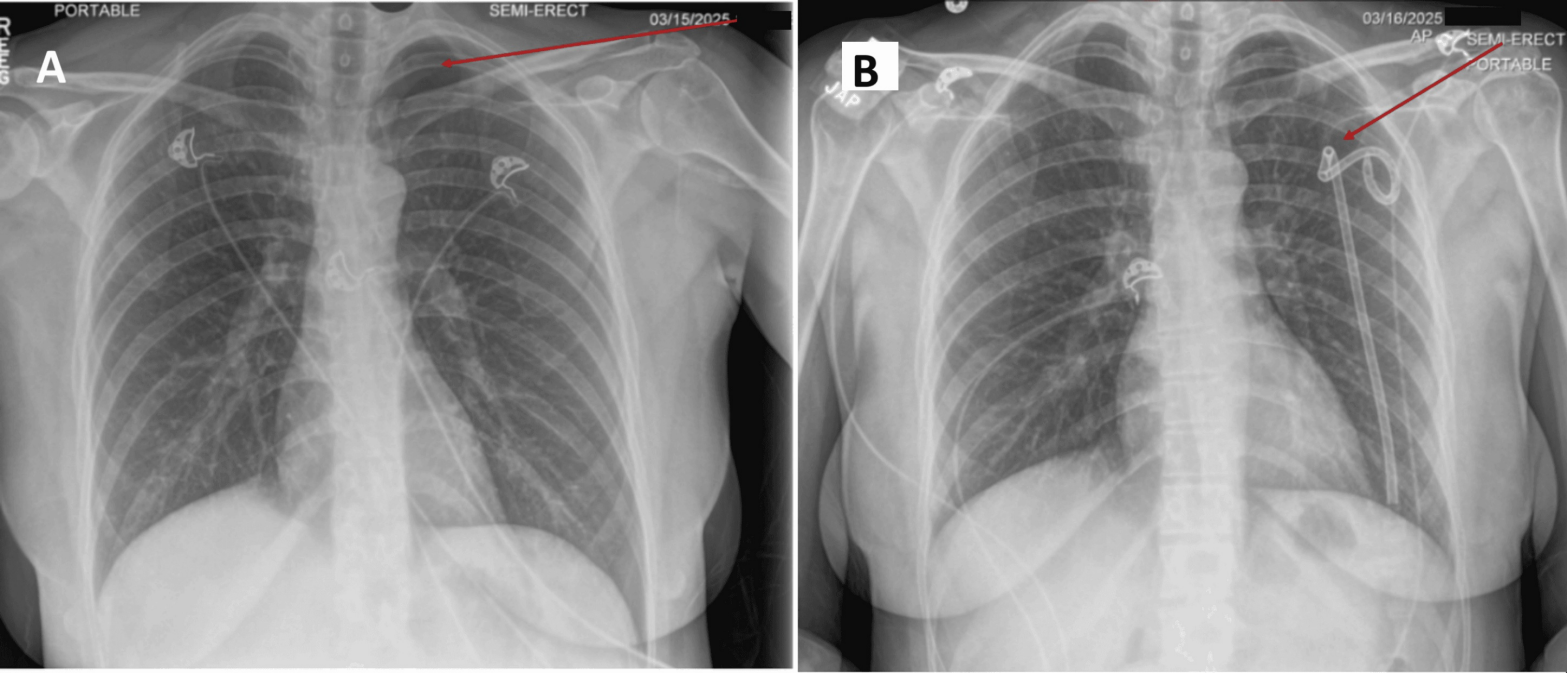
Portable chest X-ray showing (A) a left apical pneumothorax with decreased haziness on the left, and (B) the pigtail catheter that was inserted to drain the intrapleural air.
